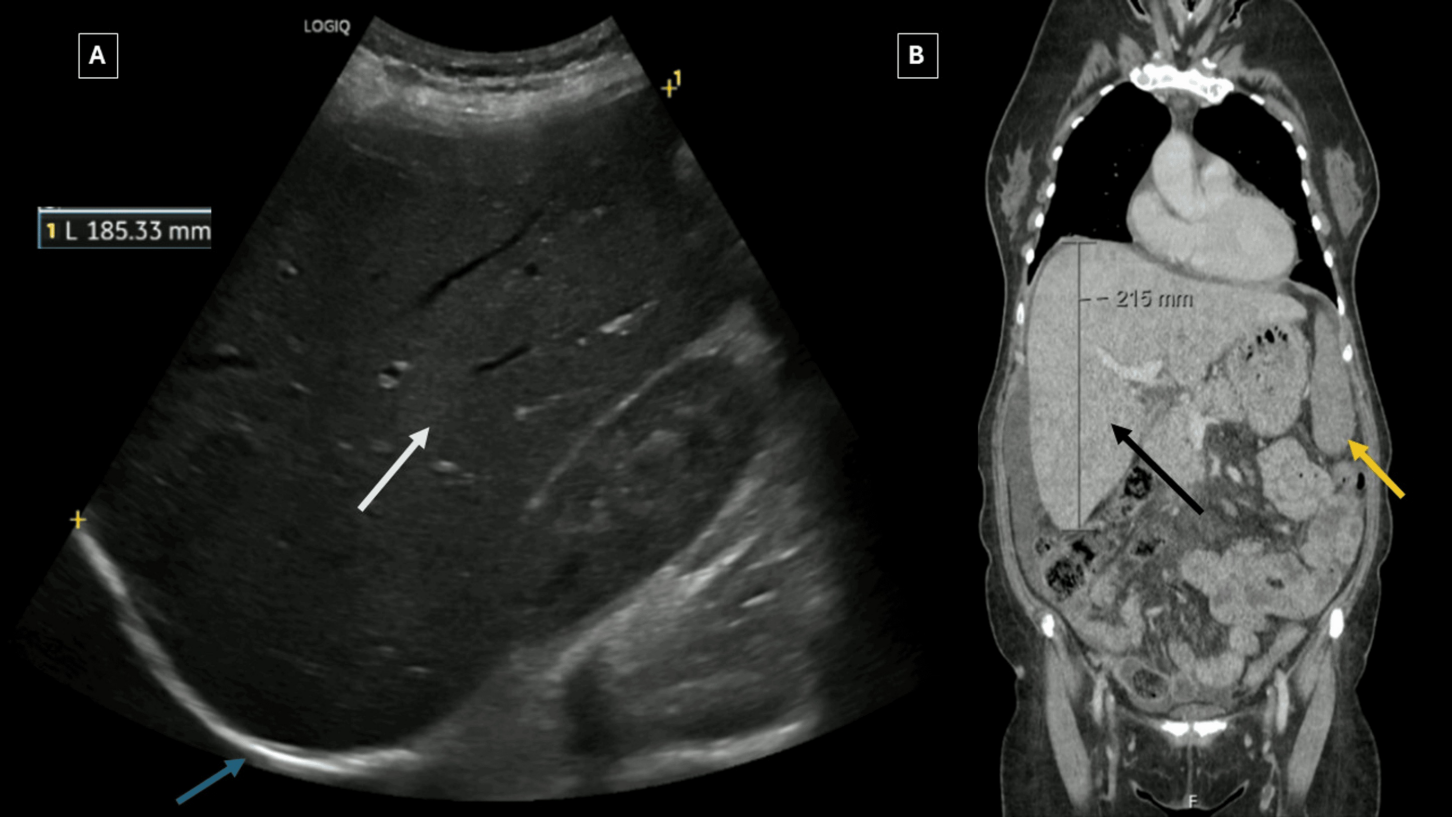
(A) Abdominal ultrasound showed hepatomegaly with homogeneous echotexture (white arrow) and rounded hepatic contours (blue arrow), raising concern for infiltrative hepatopathy. (B) Contrast-enhanced CT confirmed an enlarged liver with heterogeneous parenchymal enhancement (black arrow) and mild splenomegaly (yellow arrow).
